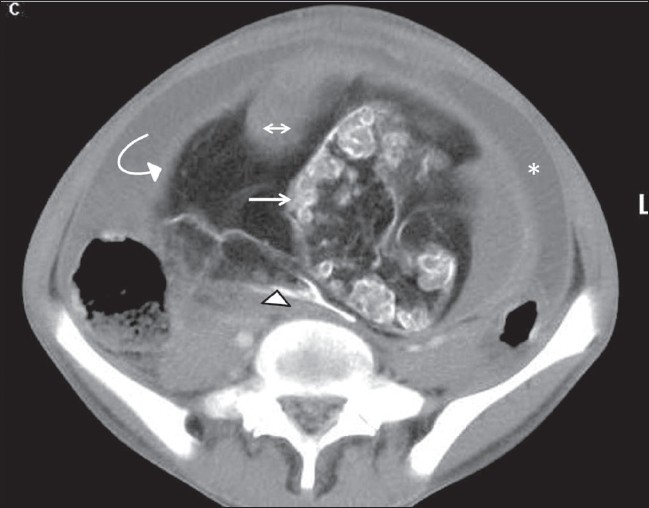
Axial contrast-enhanced CT scan shows the spine (arrow) and sacrococcyx (arrowhead) of the bigger fetus surrounded by fat and enclosed in a capsule (curved arrow). A soft-tissue plug is seen (double arrow). Ascites (*) is also seen
